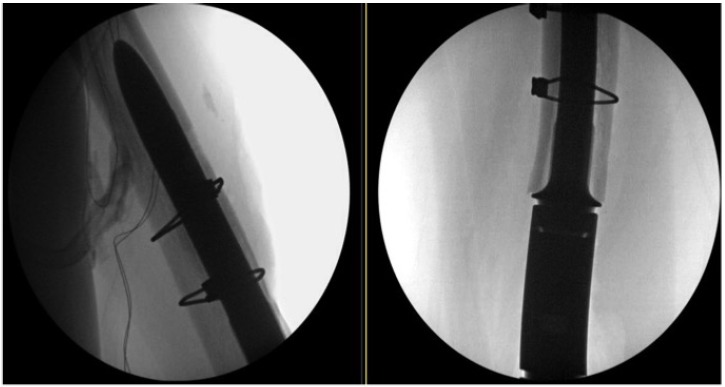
Lateral and Anteroposterior Radiographs Postoperatively.
